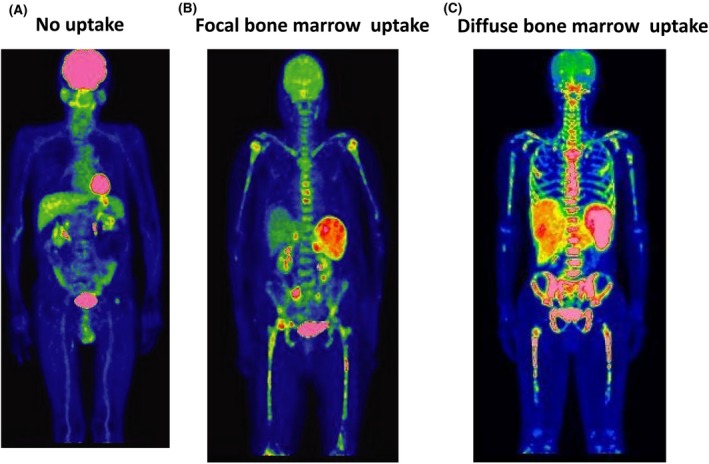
Bone marrow accumulation pattern of FDG in patients with IVLBCL. Bone marrow accumulation of fluorodeoxyglucose (FDG) was divided into three patterns*;* no uptake (A), focal (B) and diffuse (C), according to the positivity of uptake in central and appendicular bones
